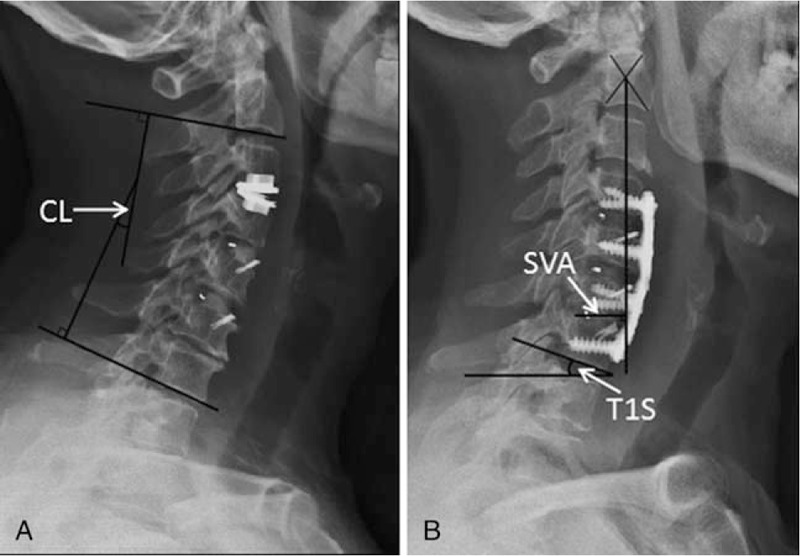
Measurements of cervical alignment parameters on standard lateral X-ray. (A) Measurement of CL; (B) measurement of SVA and T1S. CL = C2-C7 cervical lordosis, SVA = C2-C7 sagittal vertical axis, T1S = T1 slope; CL was the angle from lower endplate of C2 to lower endplate of C7, SVA was measured from C2 plumb line to posterior margin of the upper endplate of C7; T1S was from upper endplate of T1 to horizontal line.
